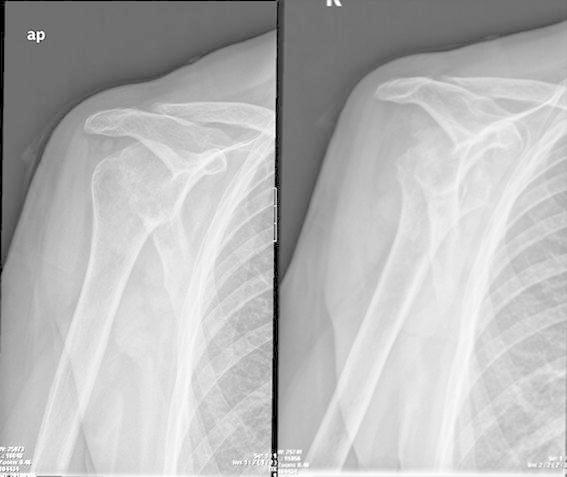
Shoulder X-ray revealing an irregular humeral head, with a bone defect on its greater tuberosity, which could correspond to the previous suture insertion
